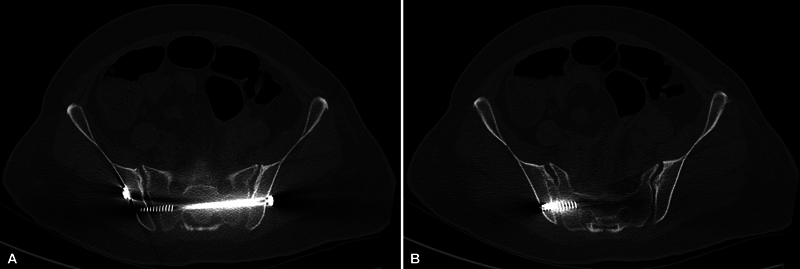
Case example (postoperative computed tomography [CT] scans). CT images demonstrating the safe and intraosseous positioning of the previously planned implant in S2.
